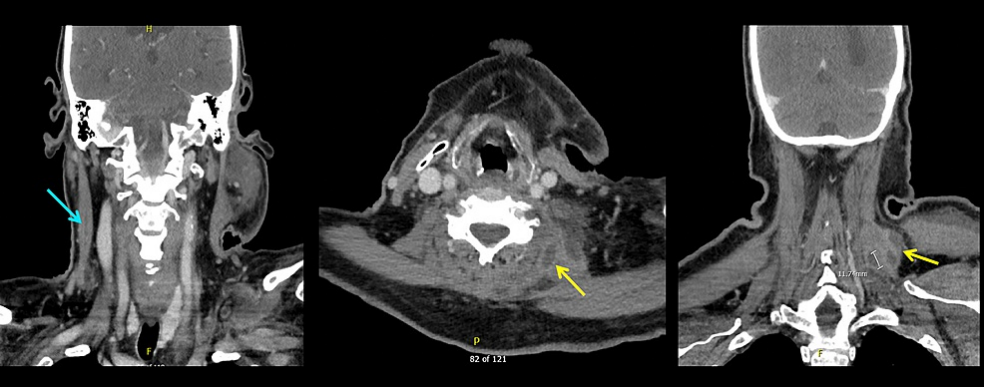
CT neck and chest imaging Blue arrow points to decreased size of right sternocleidomastoid muscle abscess. Yellow arrow points to two new left paraspinal muscle abscesses.
